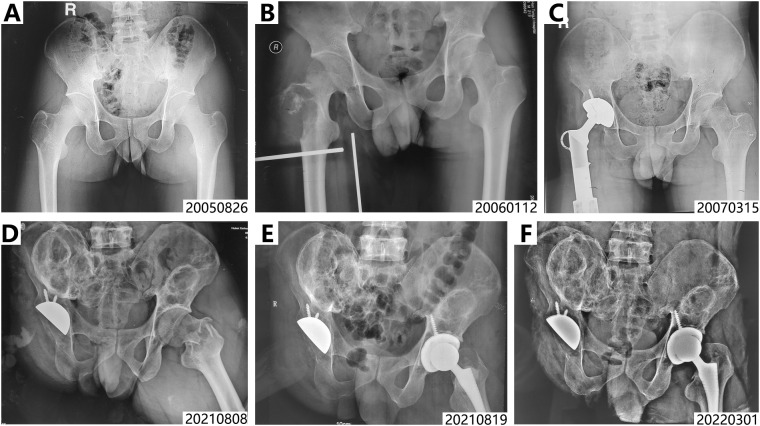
X rays of proximal femur and pelvic from Aug. 2005 to Mar. 2022. (A) The first osteolytic lesion discovered in greater trochanter of left femur in August 2005. (B) Tumor recurrence of left femur in January 2006. (C) X ray of proximal femur after right hip arthroplasty in March 2007, no osteolytic lesions were found in pelvic and left femur. (D, E) Pre- and post- hip arthroplasty X-rays from August 2021 document the management of a left intertrochanteric pathological fracture due to the discontinued therapy of denosumab. (F) Pelvic lesions manifest as more localized and circumscribed areas with increased calcification after denosumab resumption.
